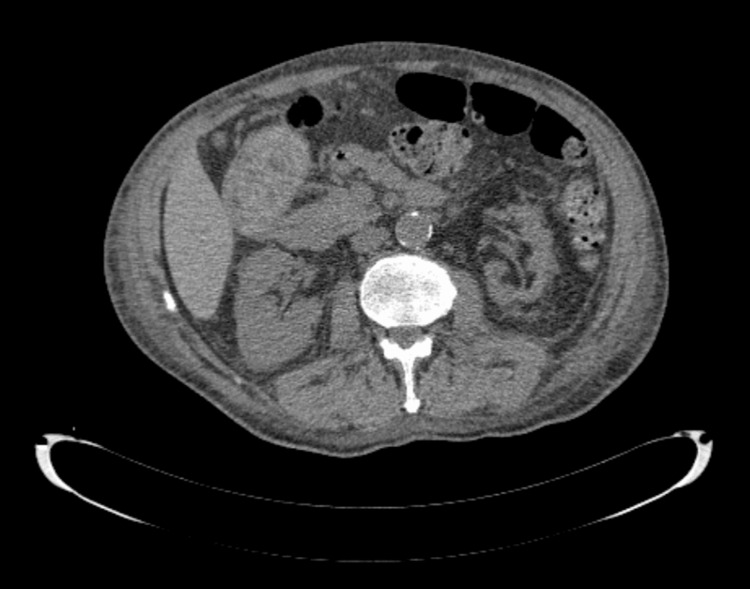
Abdominal computed tomography findings Axial abdominal computed tomography demonstrating bilateral reduction in renal cortical thickness consistent with chronic parenchymal damage.
